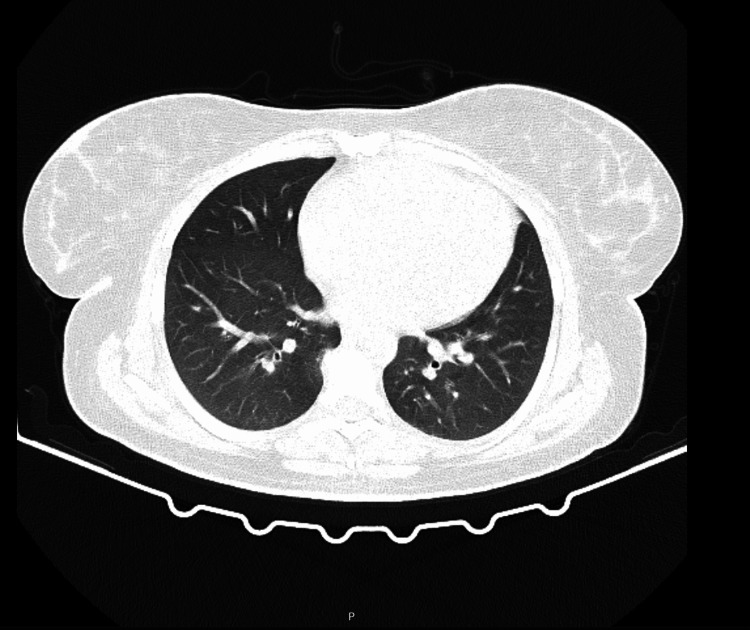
High-resolution computed tomography after 24 hours in the internal medicine ward
